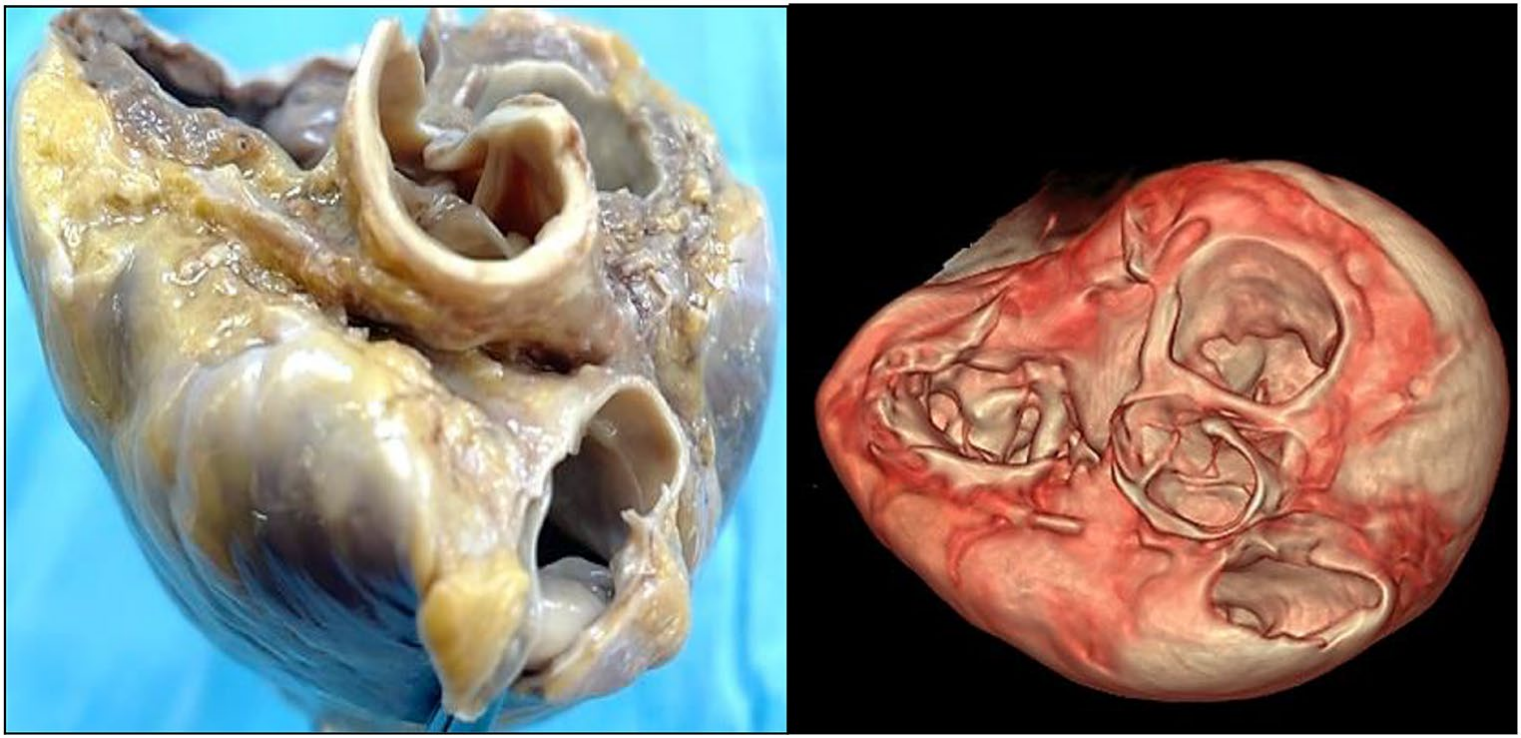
Postmortem magnetic resonance imaging (MRI) confirmed the anomalous origin and course of both coronary arteries. The right coronary artery arised form the left sinus of Valsalva, with an interarterial course between the aorta and pulmonary artery, along the atrioventricular space and subsequently in the posterior face of the heart giving the posterior interventricular artery branch. A dominant right coronary artery was present
